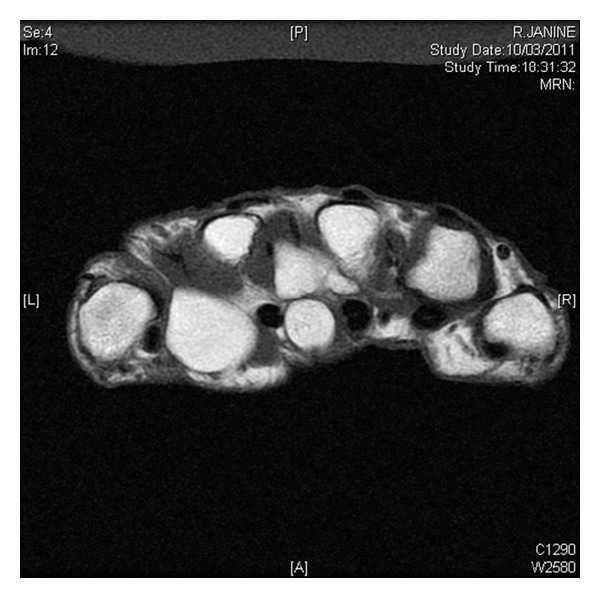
MRI axial view showing distal extension of lipoma in the first metacarpal space.
